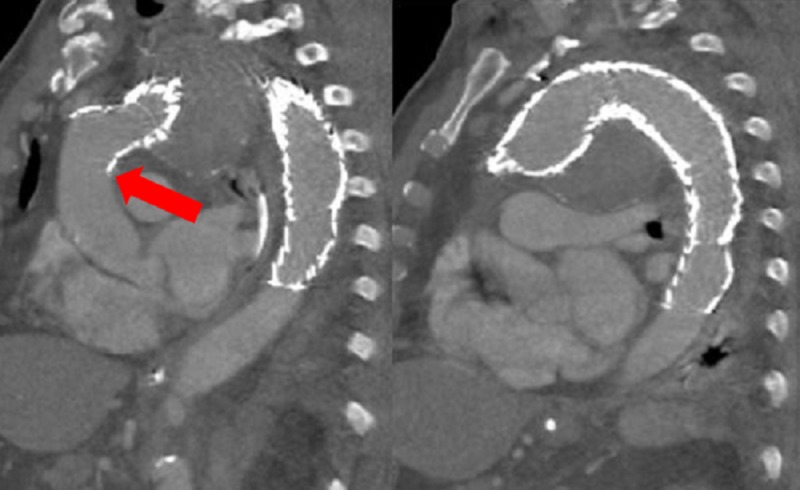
Follow-up imaging sagittal computed tomography of the chest with contrast at one-month post procedure in this patient demonstrated no complications related to stent migration, infolding or endoleak. The left image shows the proximal portion of the thoracic aortic graft (turtlehead) with no bird-beak (red arrow). The right image shows exclusion of the aneurysm with no endoleak.
